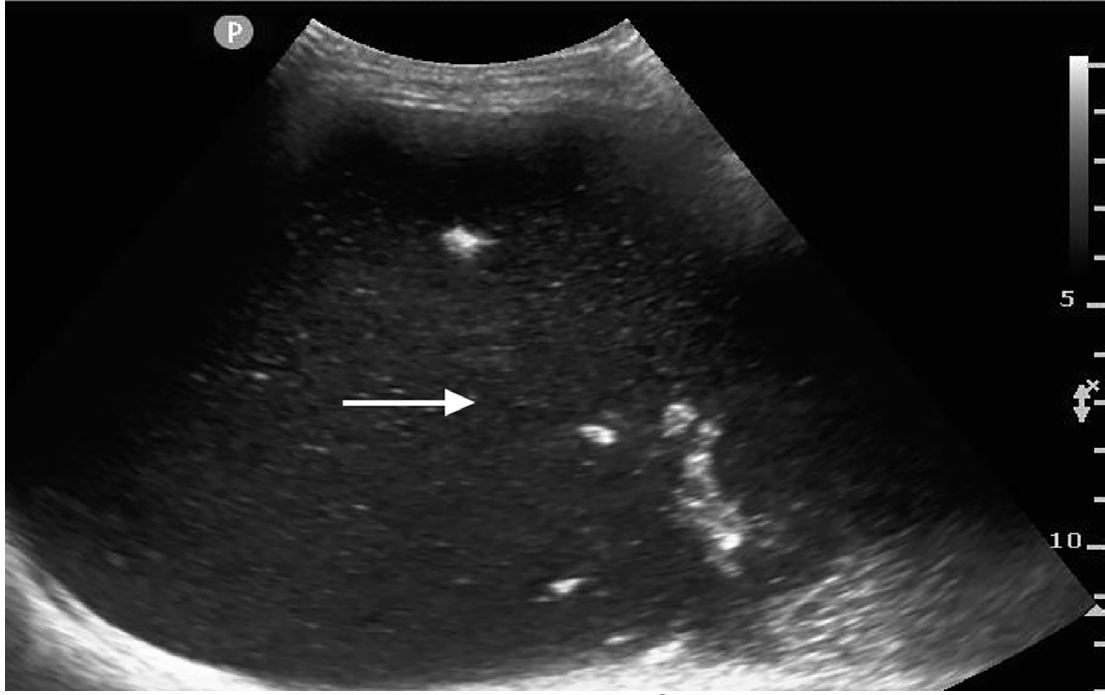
Transverse ultrasound view of abdomen demonstrating a large amount of fluid and fecal matter within a massively distended loop of bowel. Arrow is within a distended loop of bowel.
